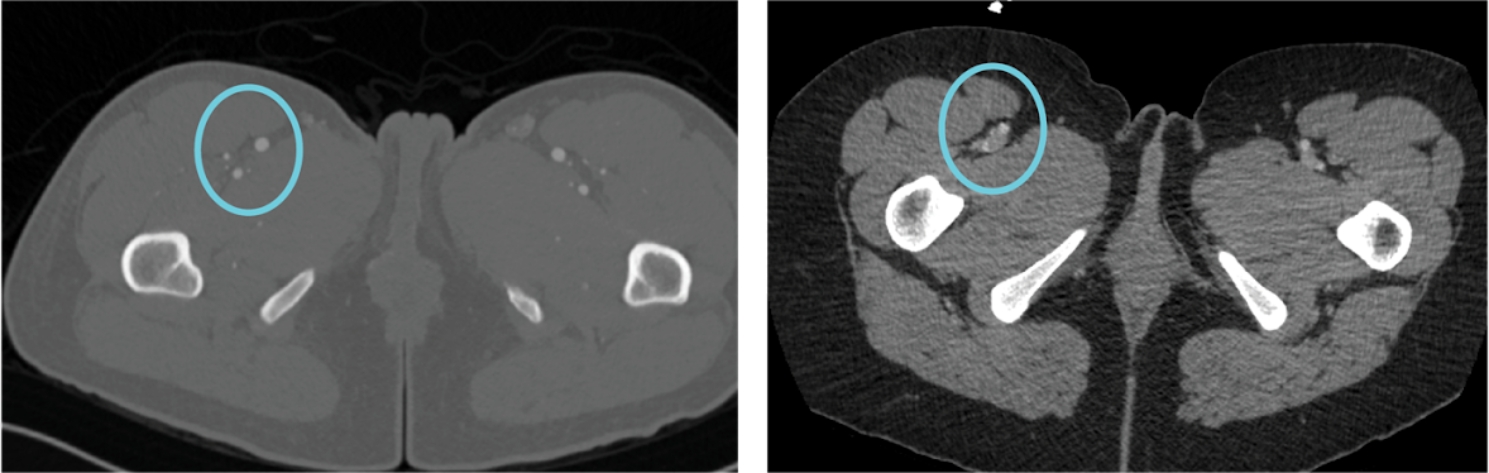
Comparison of contrasted CT Angio scan (Left) and non-contrasted CT-Scan (Right), with area inside circles displaying vasculature and their differences to the surrounding soft tissue
